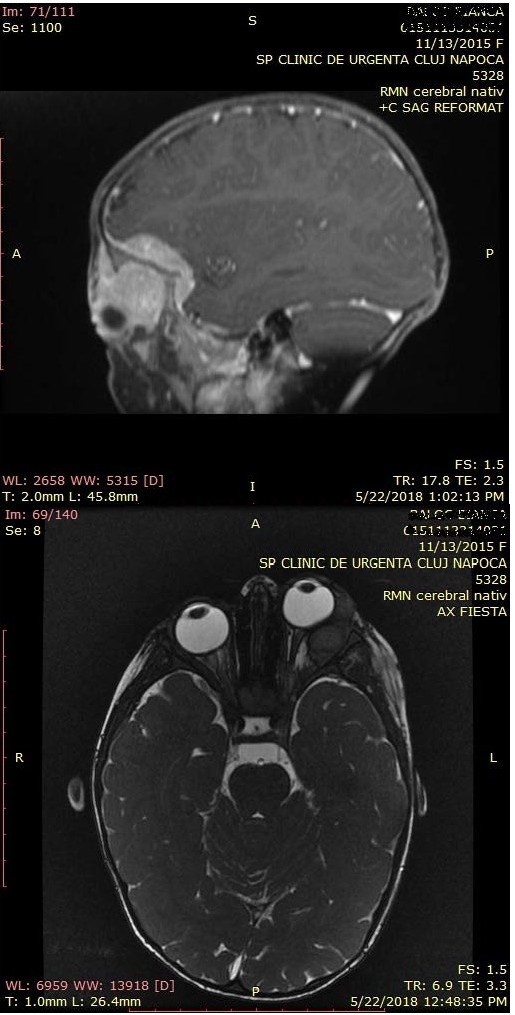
MRI of the head showing a polypoid orbital tumoral mass with extension into the left frontal, temporal, sphenoid part, into the left temporal muscle and the left frontal conjunctive tissue
